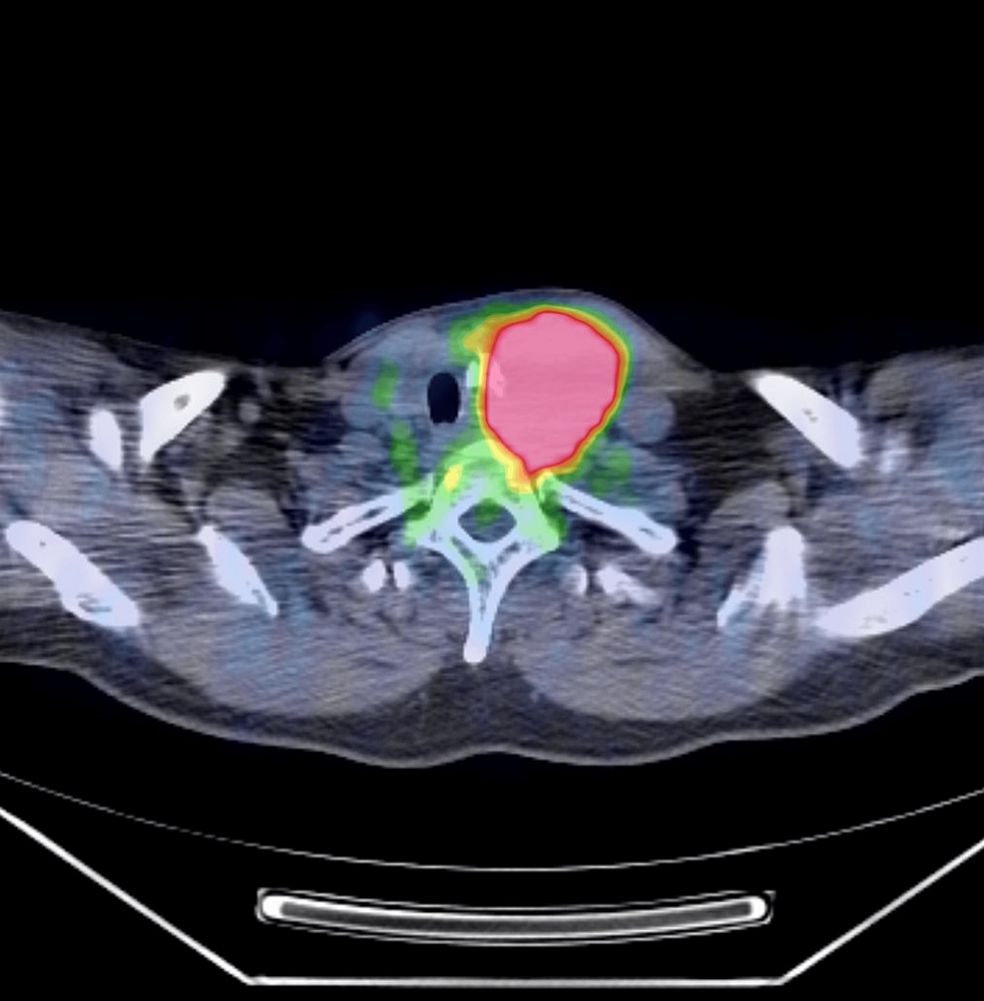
Positron emission tomography-computed tomography (PET-CT) revealed strong accumulation of 18F-fluorodeoxyglucose (18F-FDG) in the tumor, and no enlargement of regional nodes or distant metastasis was revealed.
